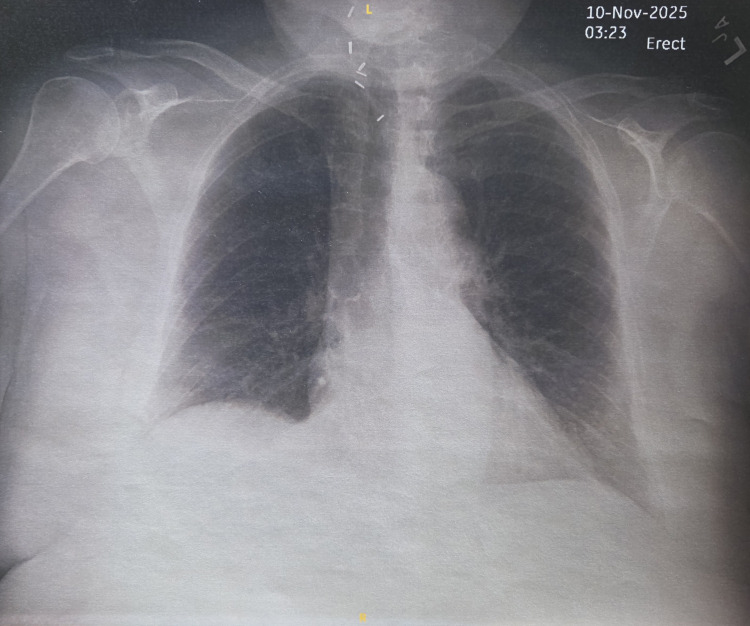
Chest radiograph demonstrating resolved right-sided pneumothorax
